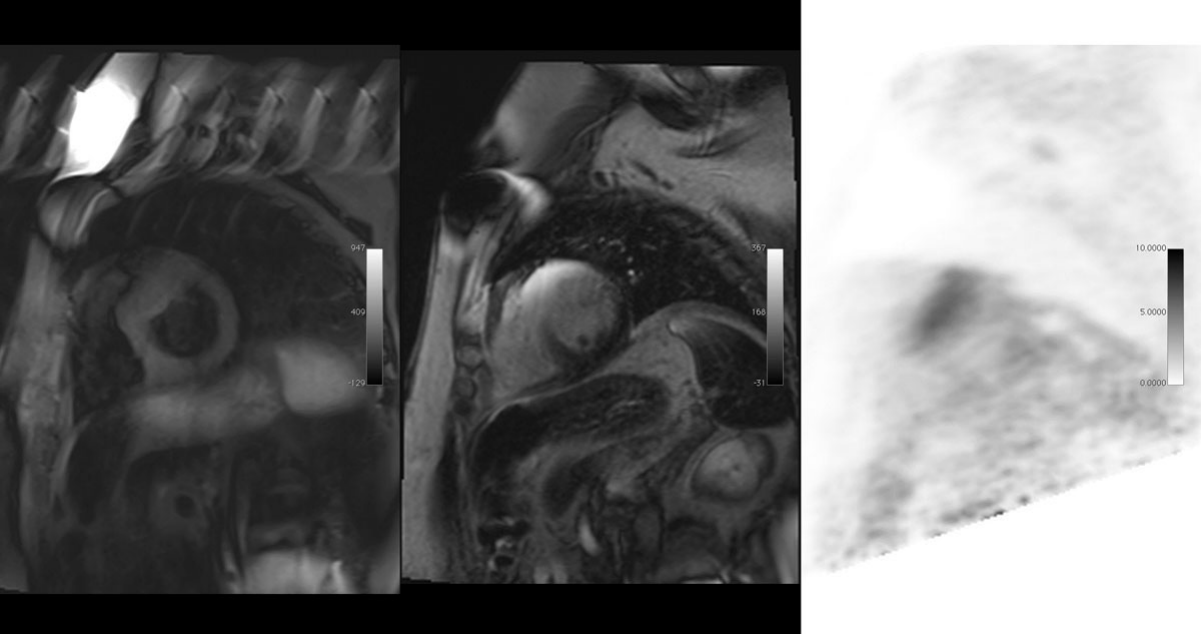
From left to right: 1) SA STIR demonstrating shows homogeneous signal from the LV myocardium. 2) SA LGE demonstrating focal mid myocardial wall LGE in the antero-septal segments. 3) SA 18F FDG PET showing focal uptake in the antero-septal segements Note is made of a Reveal device visualsied on images 1 & 2.
